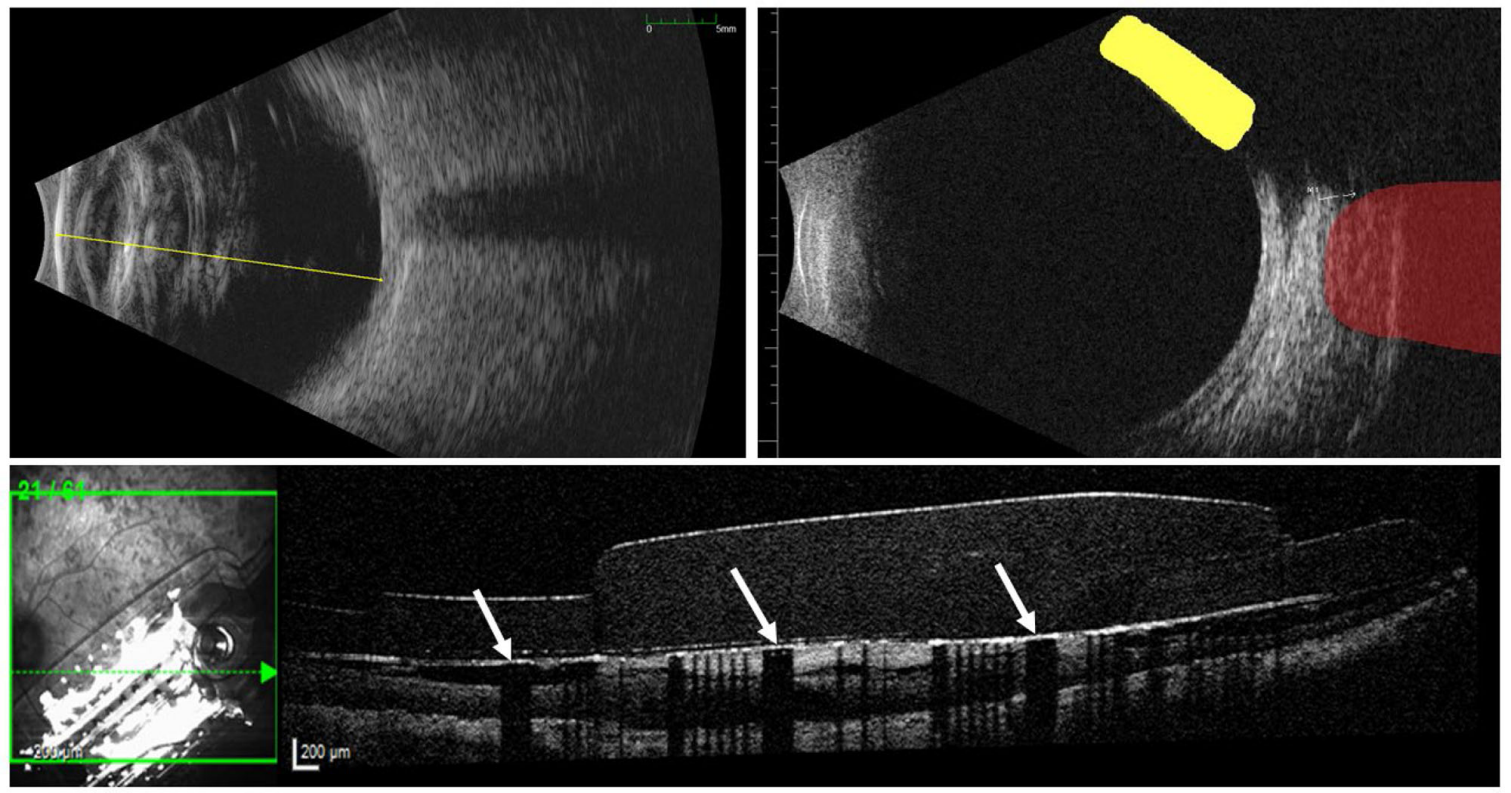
Ultrasound and OCT. The ultrasound on the top left shows axial length (yellow line). The ultrasound on the top right highlights the lateral rectus (red) and Argus EOC (yellow). The OCT scan on the bottom shows the implant, fibrotic tissue, and retinal morphology. Due to its composition, fibrotic tissue appears hyper reflective. Electrodes (white arrows) reflect light from the source, casting dark shadows on the underlying tissue.
